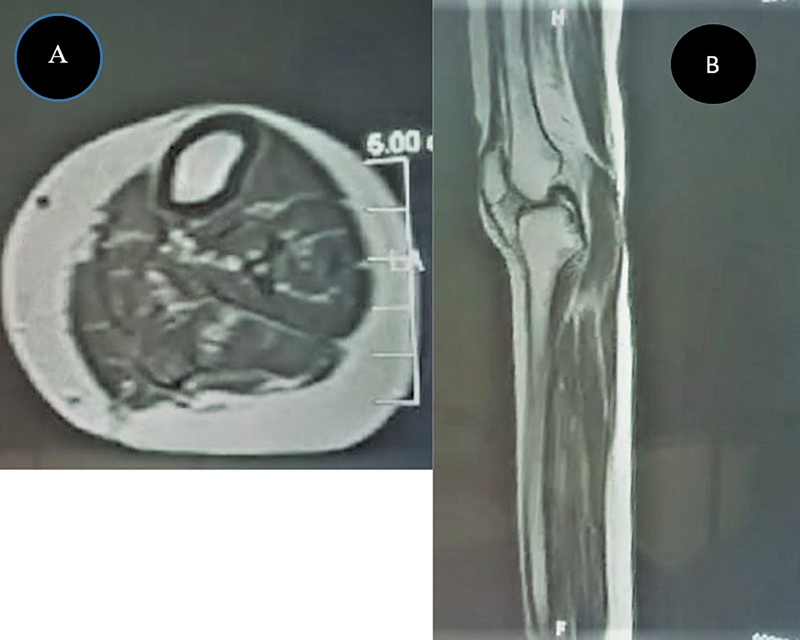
Postoperative MRI scan with contrast after open resection of a giant Baker cyst. (
